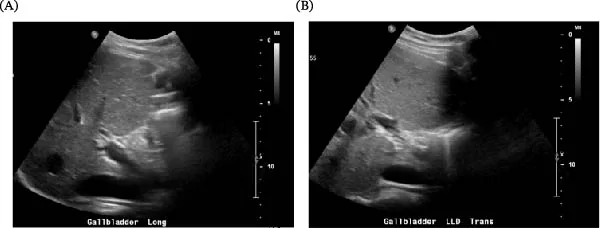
(A, B) Right upper quadrant ultrasound images with a nonvisualized gallbladder and a normal liver.
